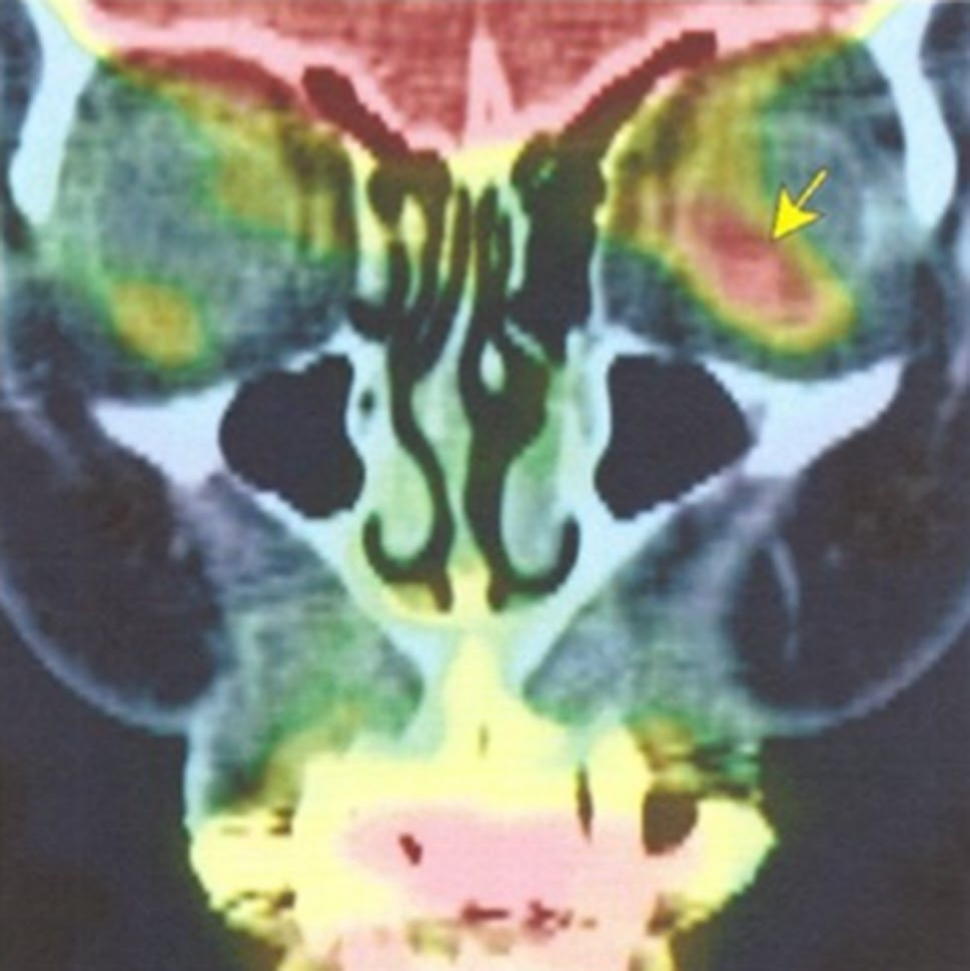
PET-CT at the time of recurrence of choroidal metastasis showed FDG accumulation in the posterior eye
